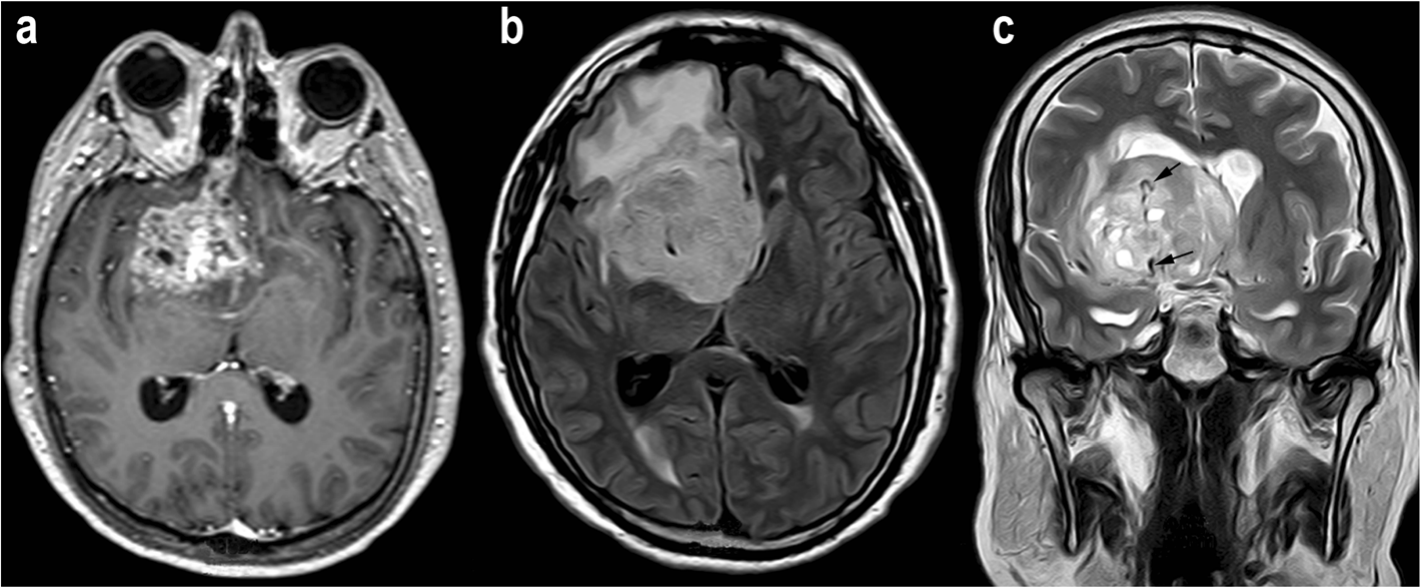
Example of MR images from a patient with glioblastoma (grade IV). a, Contrast-enhanced axial T1-weighted image shows an enhancing mass in the right frontal region. b, Axial FLAIR image shows peritumoral edema. c, Coronal turbo SE T2-weighted image revealing abnormal macroscopic vessels (arrows) within the tumor (neovascularization).
